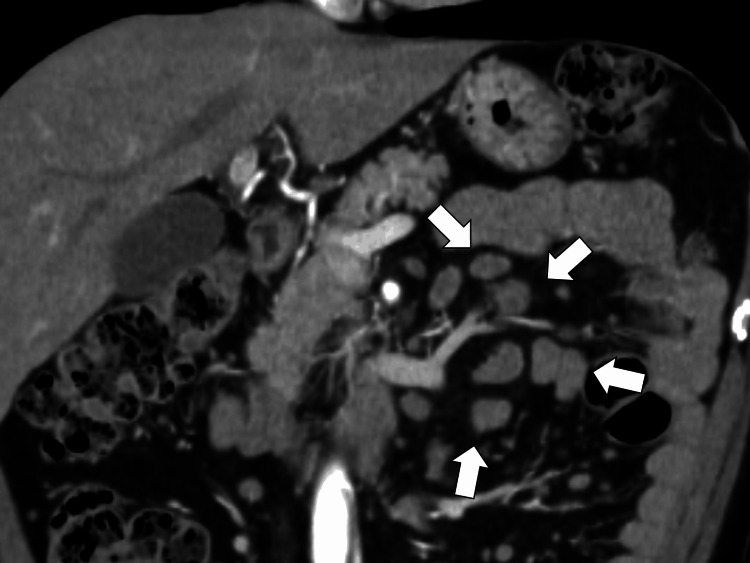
Abdominal computed tomography showing localized abdominal lymphadenopathy (white arrows)
